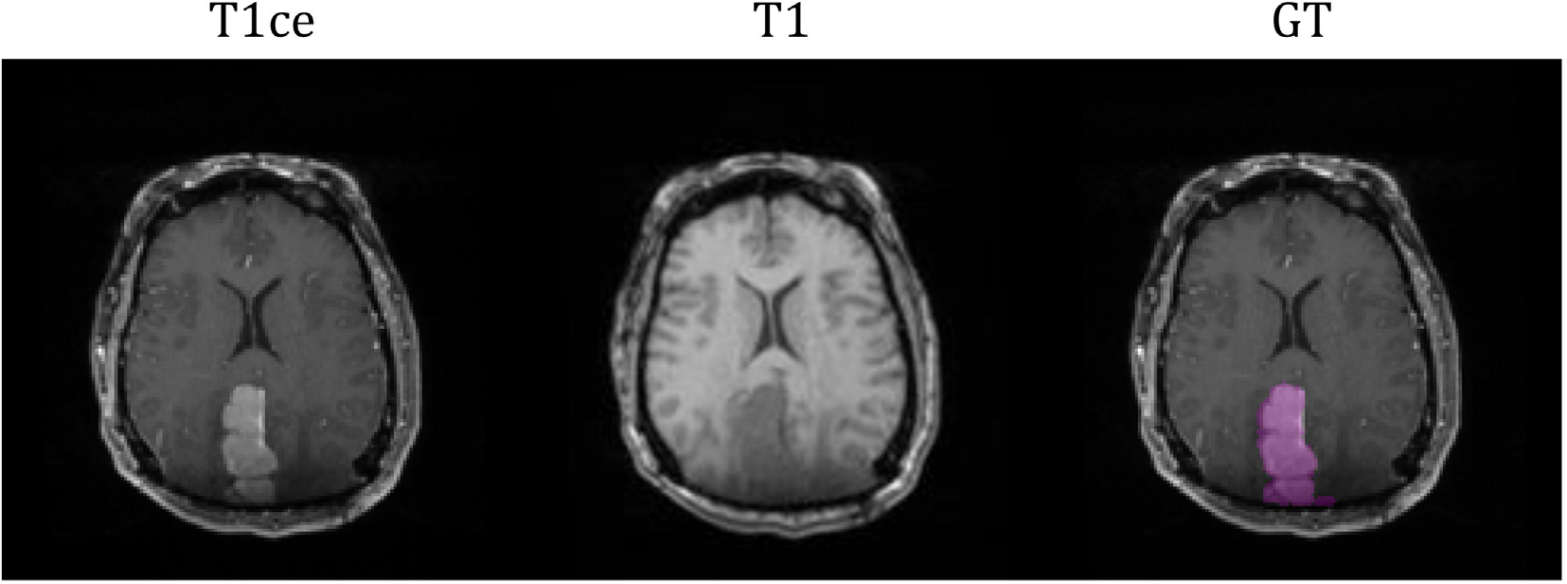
MRI sequences of T1ce and T1 with corresponding ground-truth segmentation.
